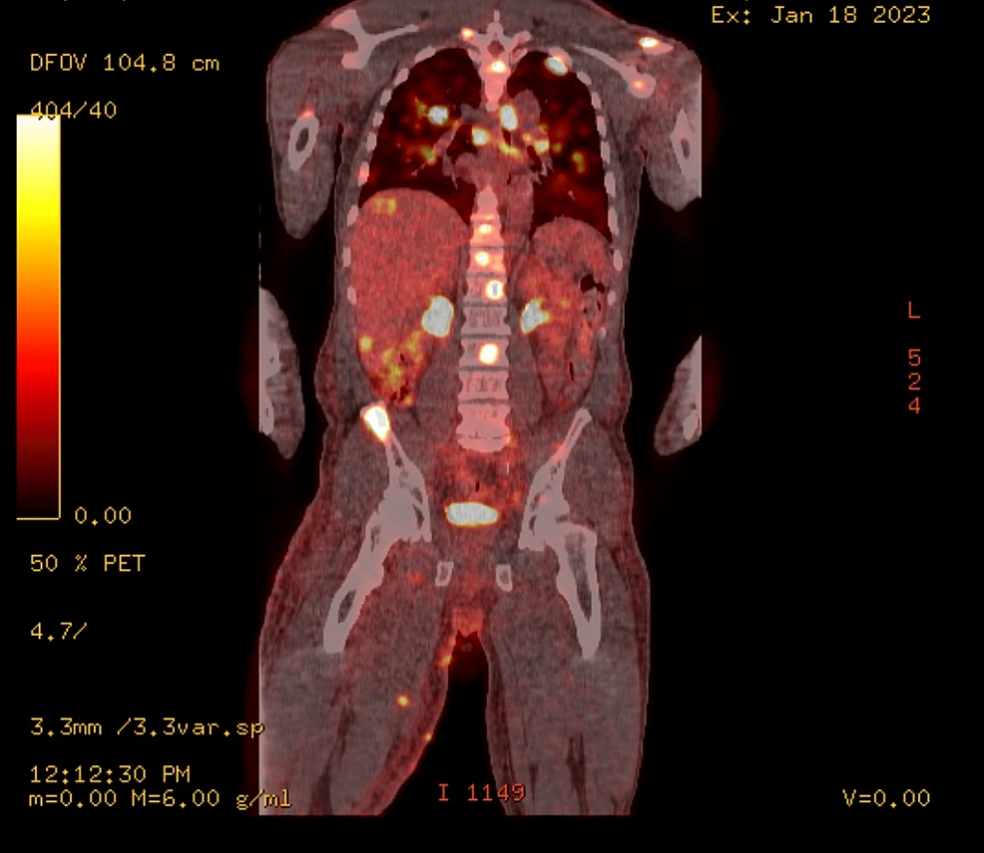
Post-chemotherapy/radiotherapy PET/CT showing new diffuse metastatic disease
